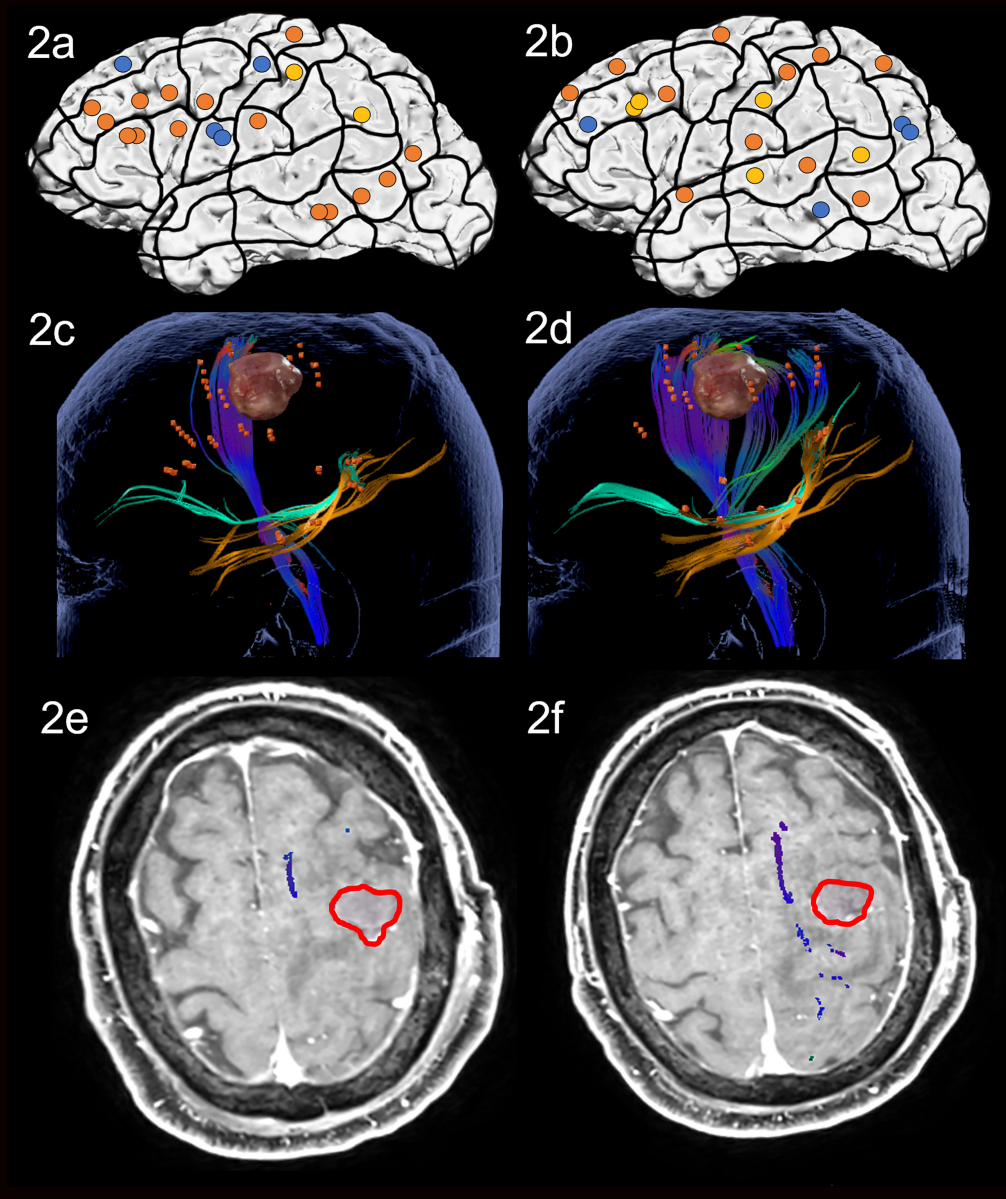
Case 2. Left panels show ON results, right panels AN results. Panels (A, B) nTMS stimulation sites that induced errors; no response and hesitations in orange, semantic errors in yellow and performance errors in blue. Panels (C, D) Fiber tracking results including the lesion, nTMS cortical endpoints in orange and color-coded tracts with CNT in direction-coded, here dark blue, IFOF in turquoise, and ILF in yellow. Panels (E, F) Overlap of tracking and postoperative T1-weighted imaging after elastic fusion with the resection cavity marked in red.
